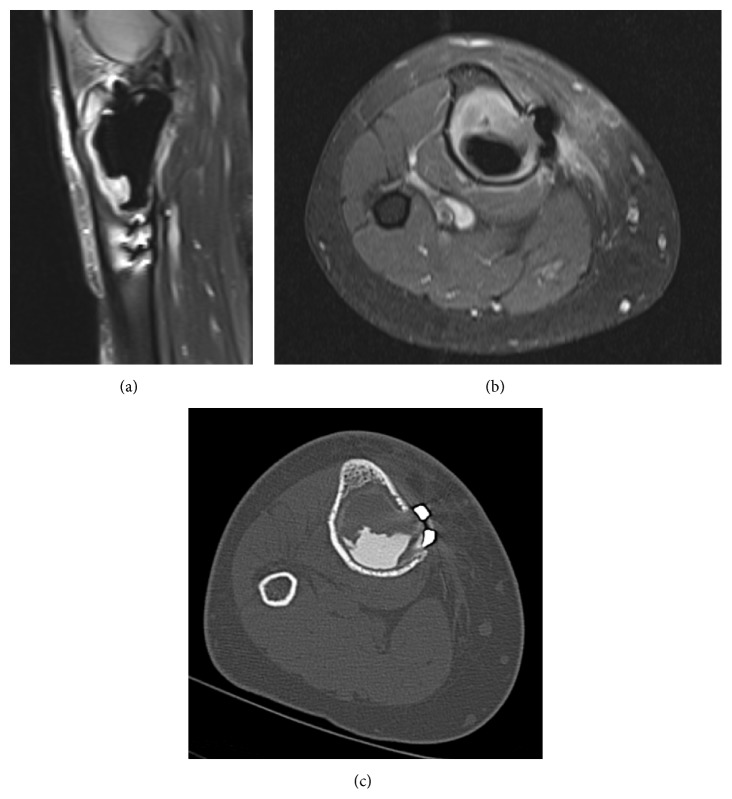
Sagittal T2 weighted fat suppressed image (a) and axial postcontrast enhanced T1 weighted image (b) of the right knee 3 months after the giant cell tumor curettage and packing demonstrate area of T2 hyperintensity and associated enhancement anterior to the surgical curettage cavity. CT scan image (c) from the same date also shows that there is corresponding uncemented area.
